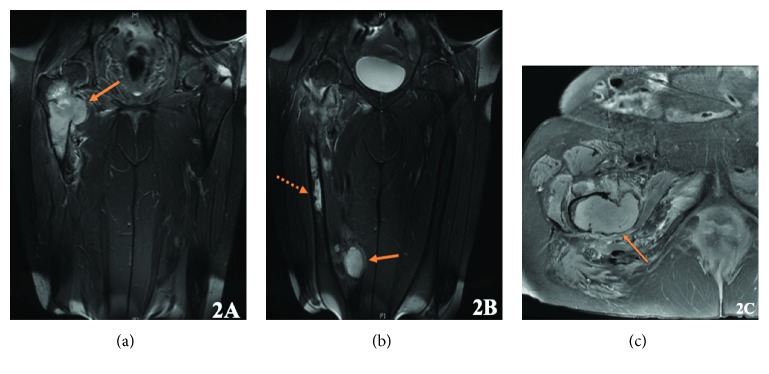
Fat-suppressed T2 MRI of the right femur. (a) Cortical destruction with an extraosseous soft-tissue mass in the region of the lesser trochanter. (b) Intermediate to high signal lesion within the medullary cavity of the proximal and mid right femoral shaft though indolent at the inferior aspect (dashed arrow). T2 hyperintense mass in the distal thigh in line with a myxoma (solid arrow).
